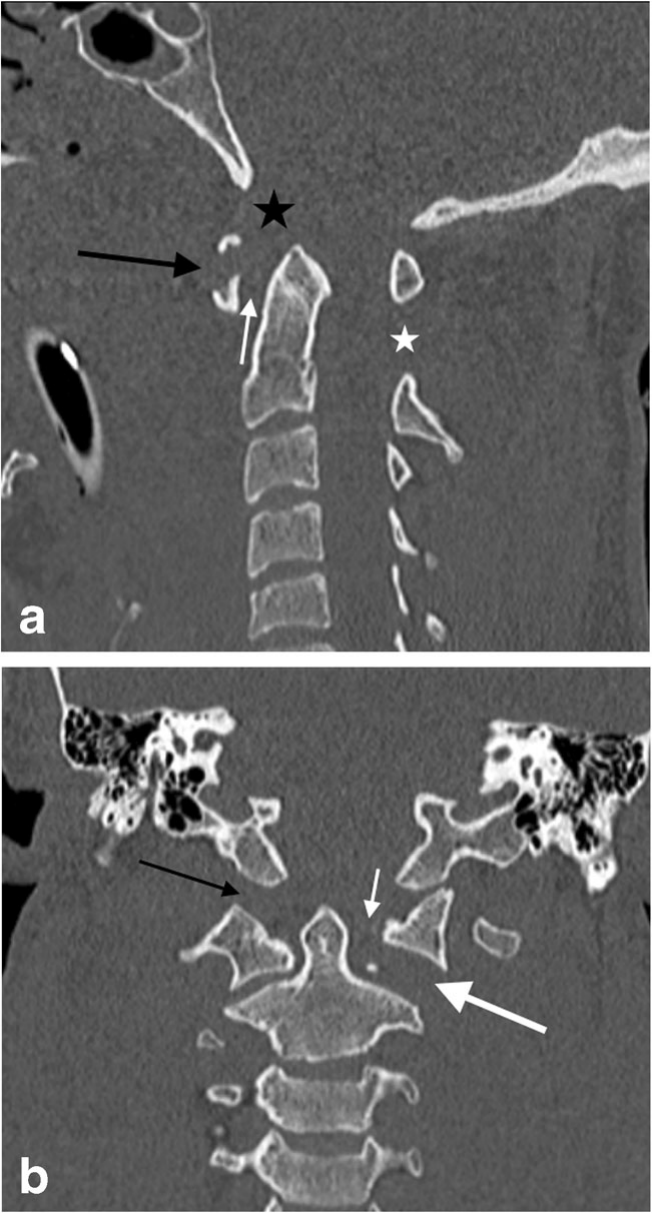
Jefferson’s fracture in a young male victim of a motor vehicle accident who suffered cardiac arrest at the scene and succumbed to his injuries shortly after presenting to the trauma centre: a sagittal reformatted CT image of the craniocervical imaging assessment demonstrates severe craniocervical junction disruption with Jefferson’s fracture (type V) of the atlas (*black arrow*) and associated abnormally widened predental (atlantodental); *white arrow*) in keeping with transverse ligament disruption and a pathologically widened basion-dens interval (*black asterisk*) in keeping with a distraction mechanism likely disrupting the critical alar ligaments, cruciform ligament (vertical band) and probably the tectorial membrane to some degree as well as the C1-C2 components of the ligamentum nuchae and flavum (*white asterisk*); b coronal reformatted CT image of the same patient confirms type II atlanto-occipital distraction (*black arrow*) associated with Jefferson’s fracture and disruption of the atlantoaxial joint on the left (*large white arrow*); the left atlantodental interval is abnormally widened (*small white arrow*) associated with Jefferson’s fracture of the atlas
